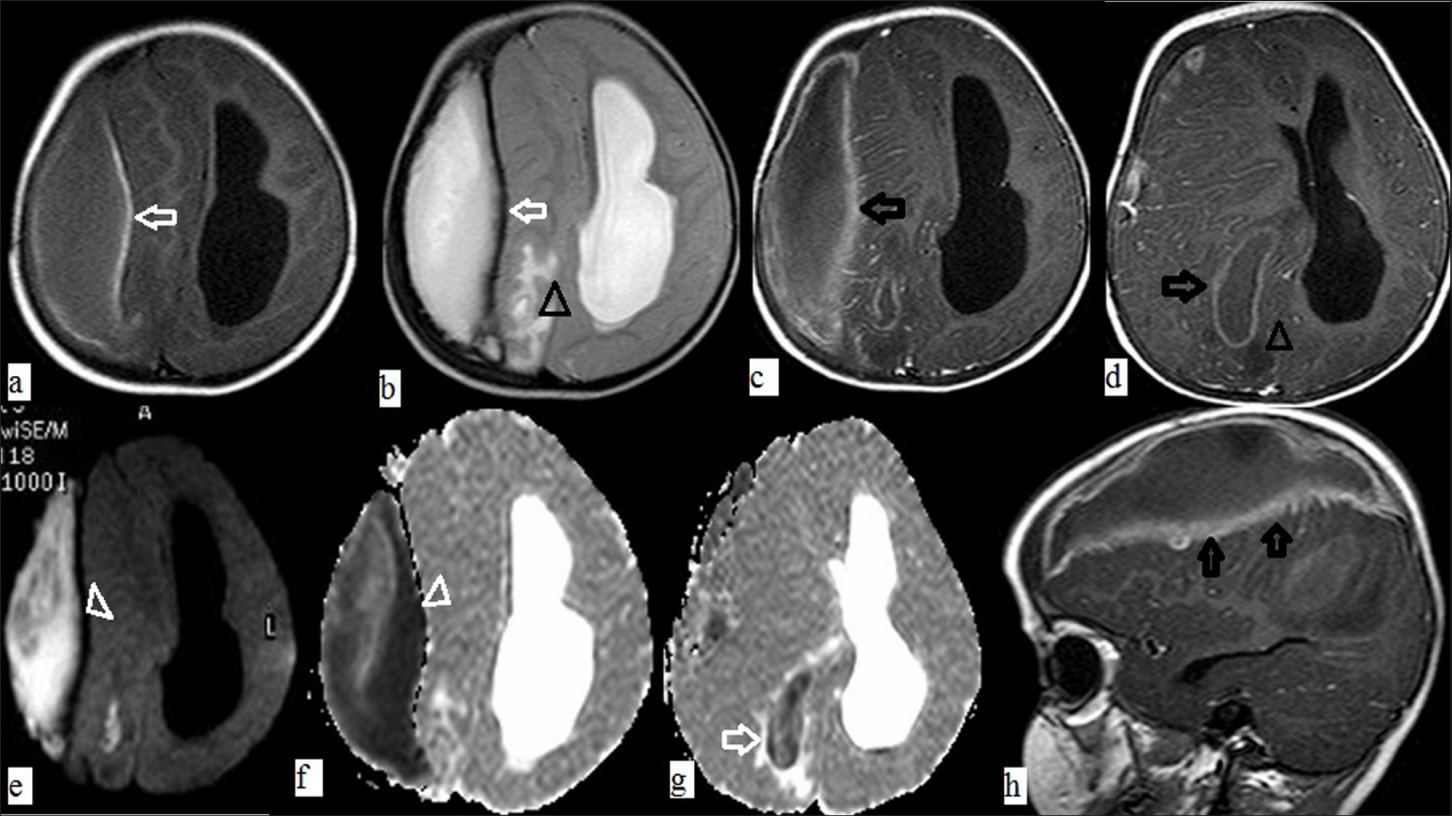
On MR imaging, walls of the subdural collection are hyperintense on noncontrast axial T1- (a, white arrow) and hypointense on axial T2-weighted (b, white arrow) images compatible with calcification. On isotropic b 1000 image (e) and ADC maps (f, g) restricted diffusion in the infected areas (white arrowheads) as well as in the occipital horn of the lateral ventricle (image g, white arrow), consistent with pus accumulation were observed. On contrast enhanced axial (c, d) and sagittal T1-weighted (h) images, abscess wall was irregularly enhancing and increased ependymal enhancement within the lateral ventricles as well as of the overlying meninges are observed (black arrows). Finally, intraparenchymal and intraventricular extension with surrounding vasogenic edema can be seen (black arrowheads).
